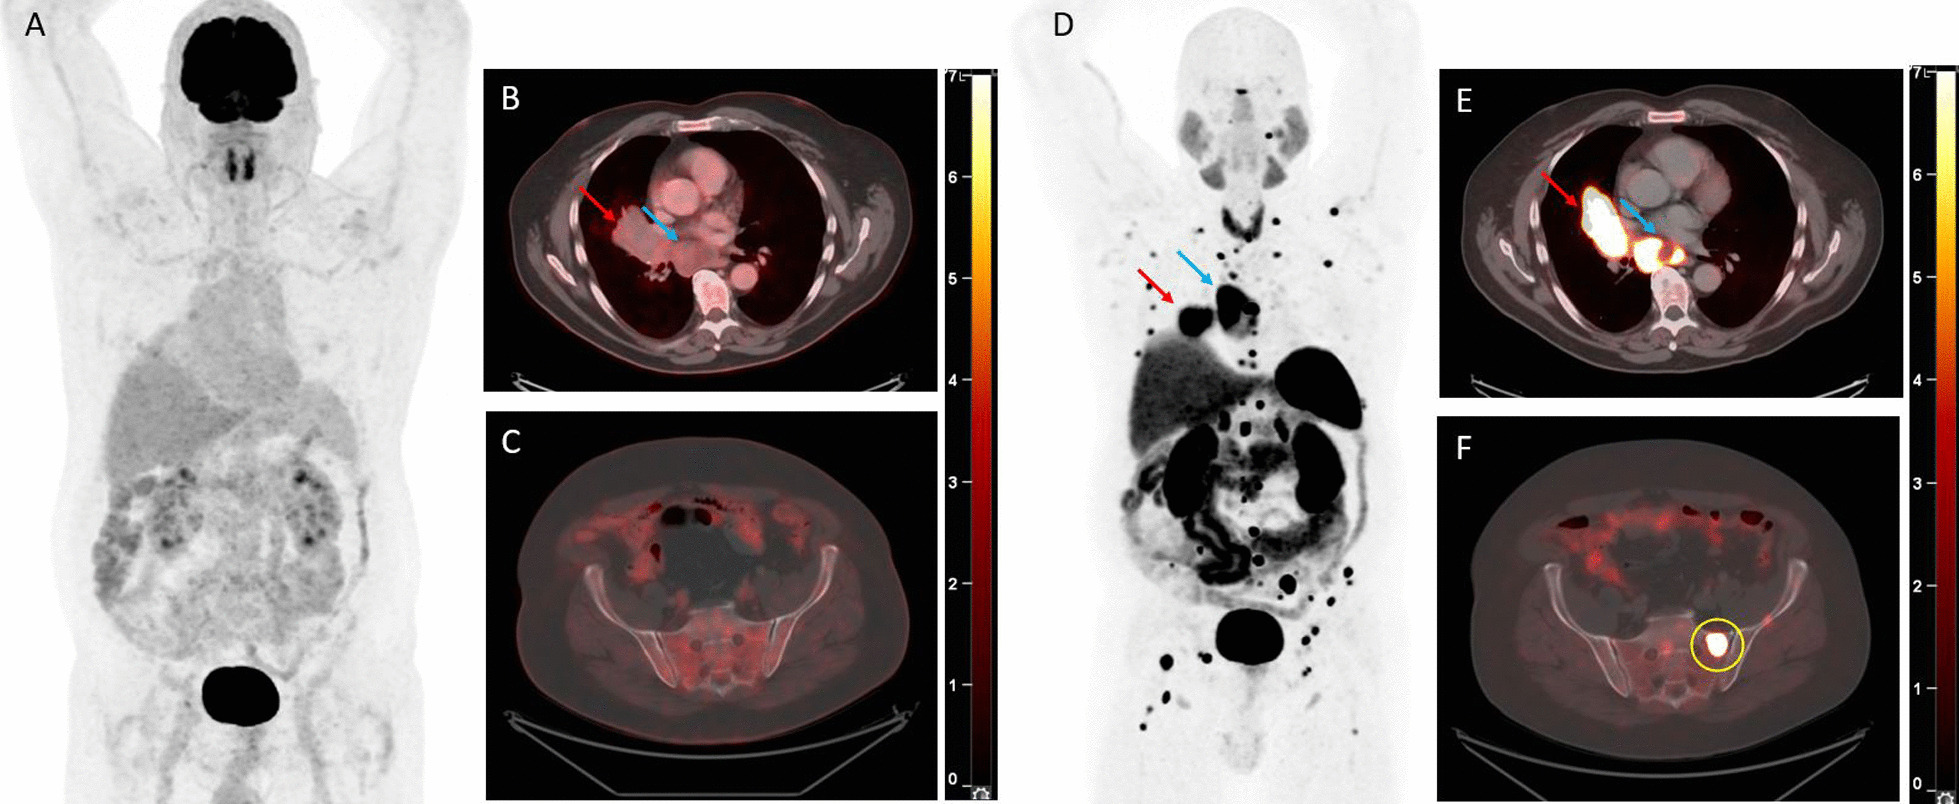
MIP image of an [^18^F]FDG PET/CT scan (A) and a [^68^Ga]Ga-DOTATATE PET/CT scan (D) performed during staging of a typical bronchial carcinoid tumour (TC) of a 62-year-old patient with a clear discrepancy between an intense [^68^Ga]Ga-DOTATATE uptake (E) and no increased [^18^F]FDG uptake (B) in the primary tumour (red arrow) as well as in a subcarinal lymph node metastasis (blue arrow). The axial fusion images with CT in bone window of the [^18^F]FDG PET/CT (C) and the [^68^Ga]Ga-DOTATATE PET/CT (F) also show this discrepancy with regard to the bone metastases. The bone metastasis in the left hemisacrum (yellow circle) has a SUV_max_ on [^68^Ga]Ga-DOTATATE PET/CT of 39.6. A chest CT 53 days before the [^68^Ga]Ga-DOTATATE PET/CT did not show any bone metastasis
